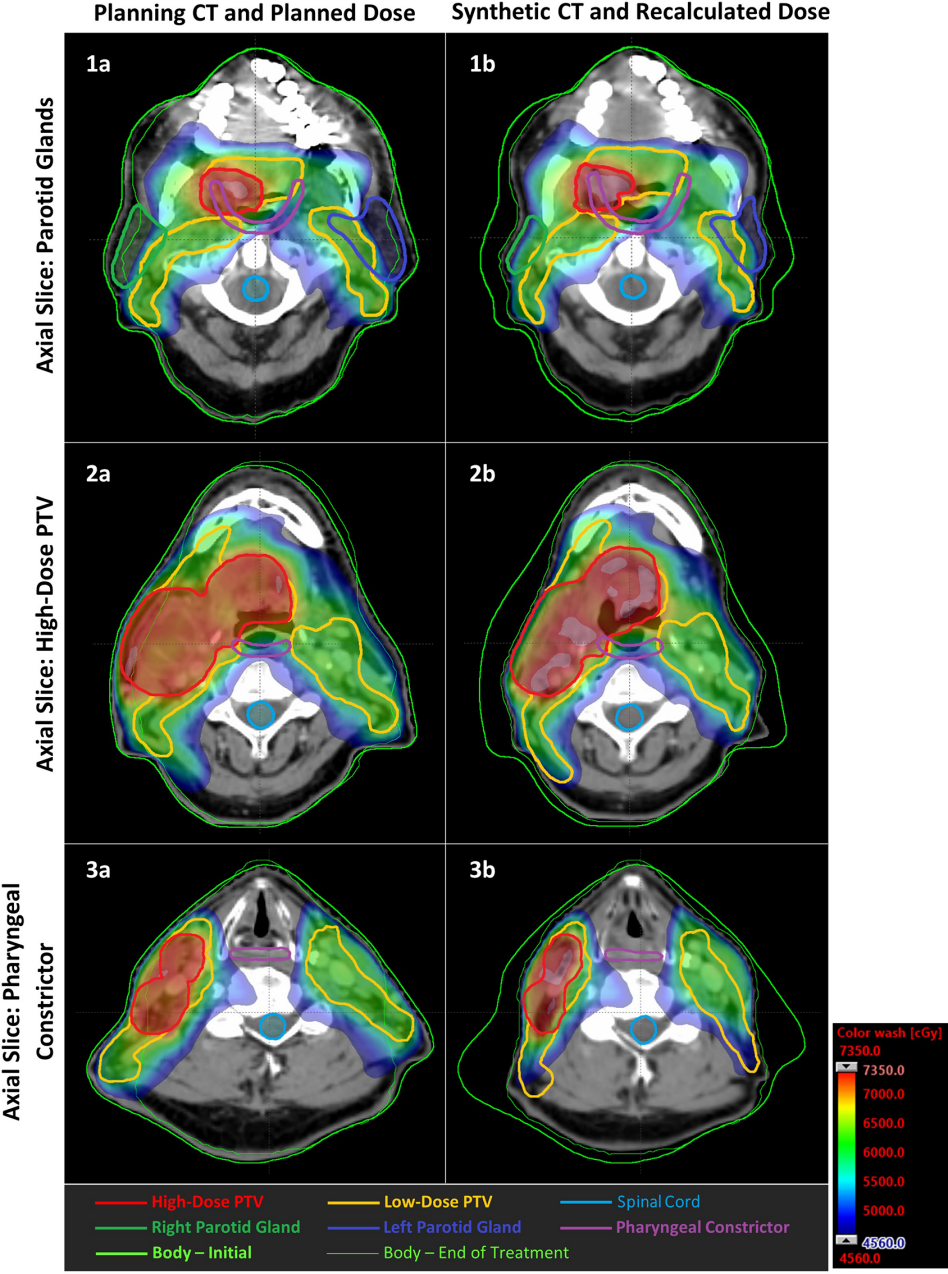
Example of the changes in patient geometry and dosimetry between the planning CT (A: left column) and synthetic CT (B: right column), here assessed at fraction 31 of 33. The patient shown was identified as having changes representative of approximately 12% of the training cohort, according to data clustering performed for deformable image registration quality assurance. Axial slices correspond to: 1) the centers of mass of the parotid glands; 2) centre of mass of the high-dose PTV; 3) centre of mass of the pharyngeal constrictor, assessed for the planning CT and rigid alignment of the synthetic CT. A dose color wash indicates doses ranging from 95% of the maximum allowable spinal cord dose, to 105% of the high-dose prescription. Anatomical structure contours are overlaid. Notably, the patient experienced weight loss, loss of parotid gland volume, and a general increase in doses to healthy tissues.
